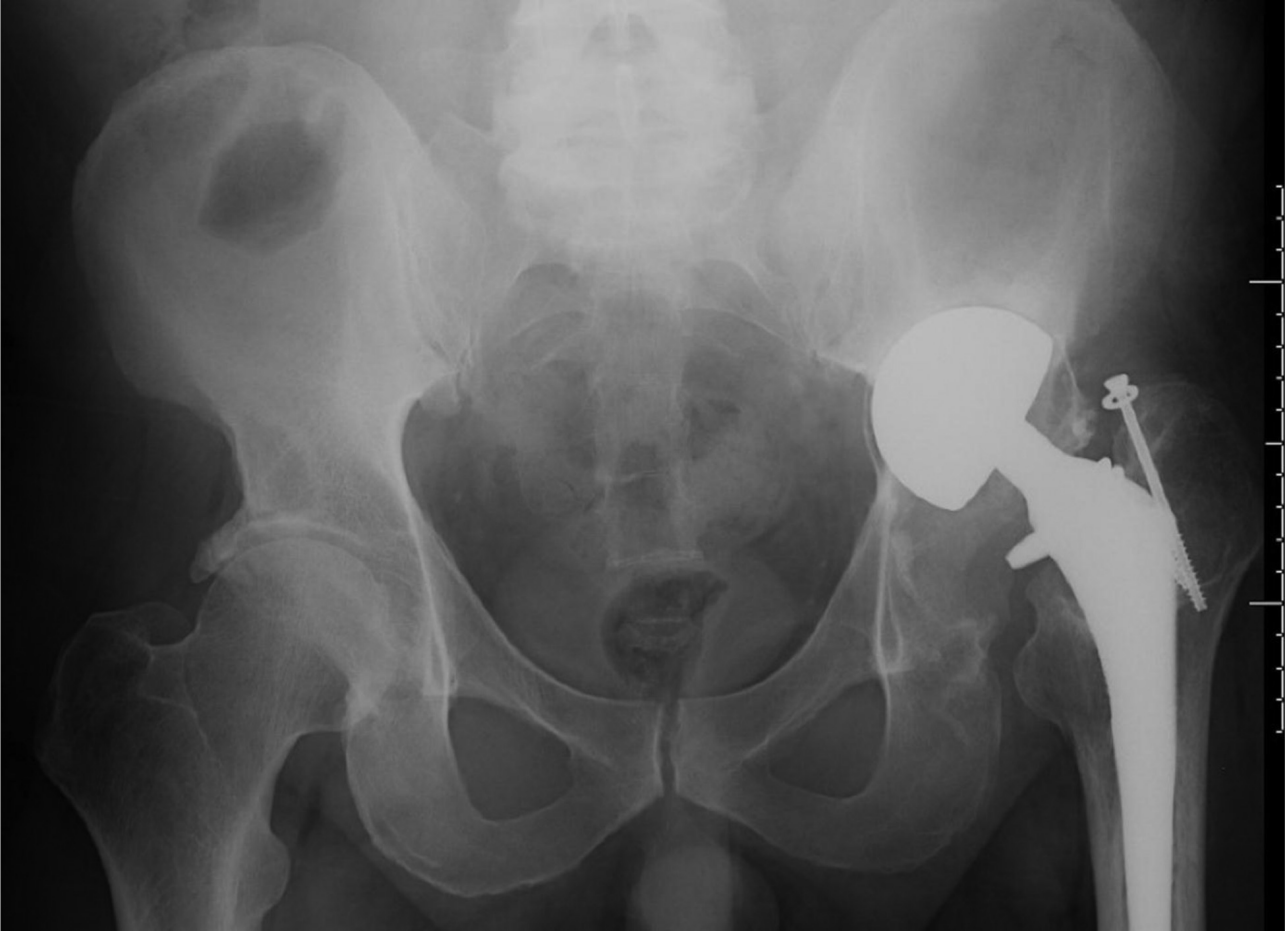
Anteroposterior radiograph of the pelvis showing a hemiarthroplasty 14 years after surgery.
